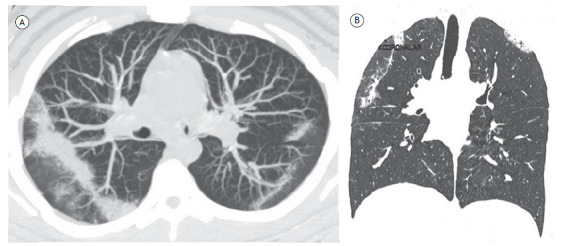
In A, axial chest CT scan showing band-like pulmonary consolidations, longitudinal to the pleural surface, in the upper lobes, predominantly on the right. In B, coronal reconstruction demonstrating the extent of the consolidations.
